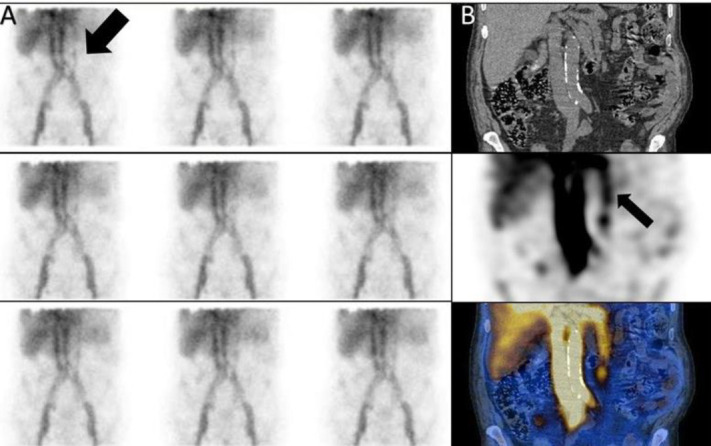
Superior mesenteric vessels on planar (A) and SPECT/CT (B)
